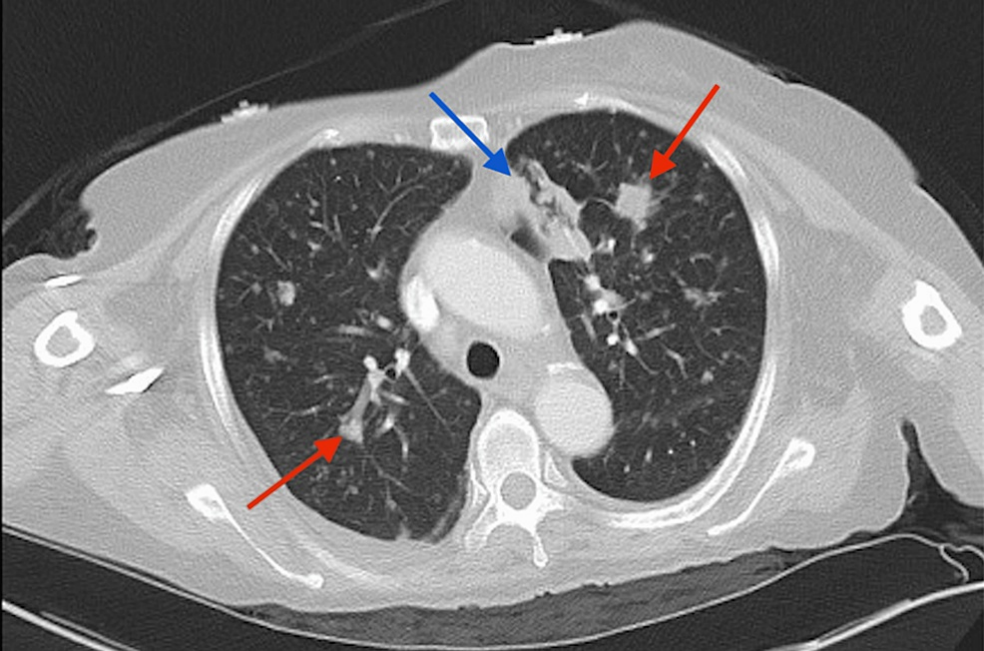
Computed tomography of the chest on day 2 of admission demonstrating bilateral spiculated ground glass pulmonary opacities (red arrows), pleural thickening of the left upper lobe pericardiac region (blue arrows), and mediastinal lymphadenopathy.
